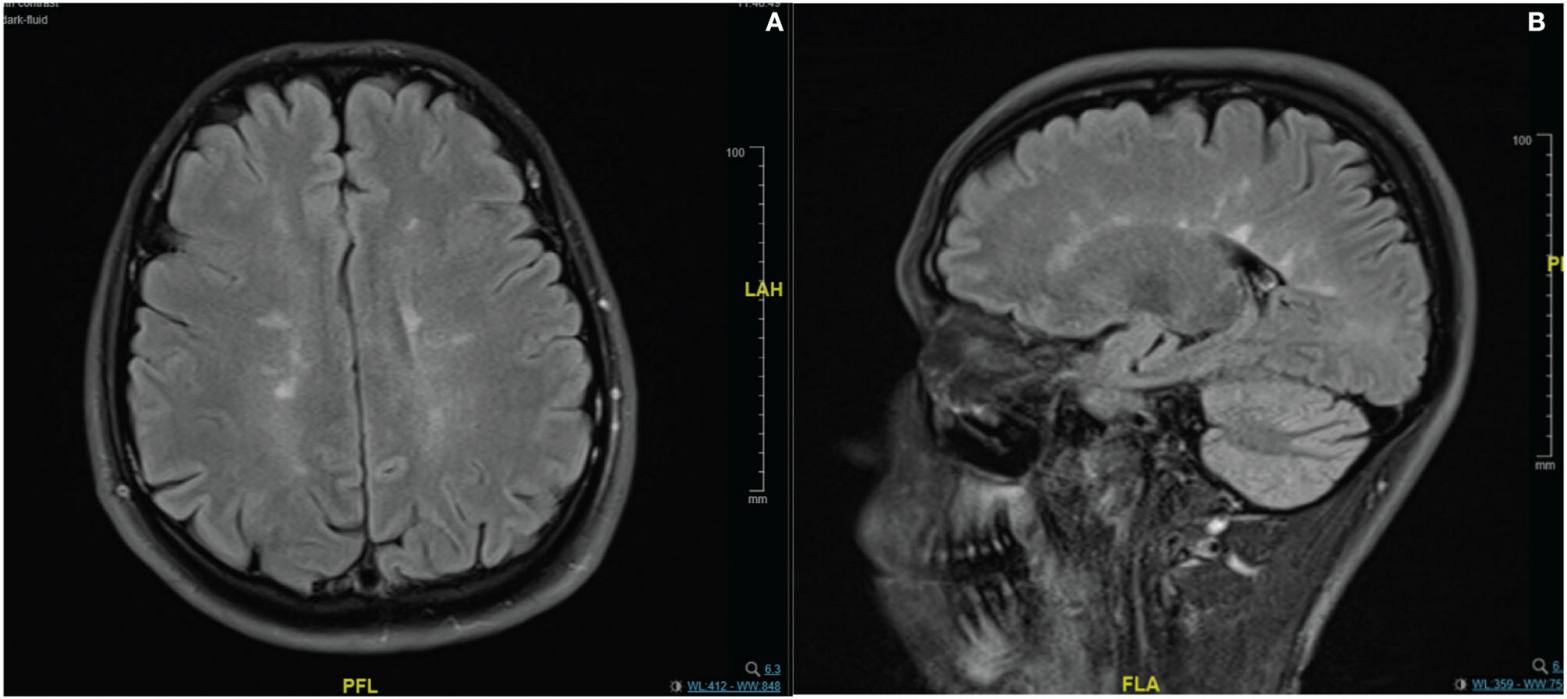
Contrast enhanced MRI brain imaging on the day of admission. Fluid attenuated inversion recovery (FLAIR) sequence (A,B). Showing multiple abnormal high signal intensities involving cortical, juxtacortical, subcortical, and periventricular regions. Some lesions were perpendicularly oriented to the corpus callosum representing Dawson's fingers.
